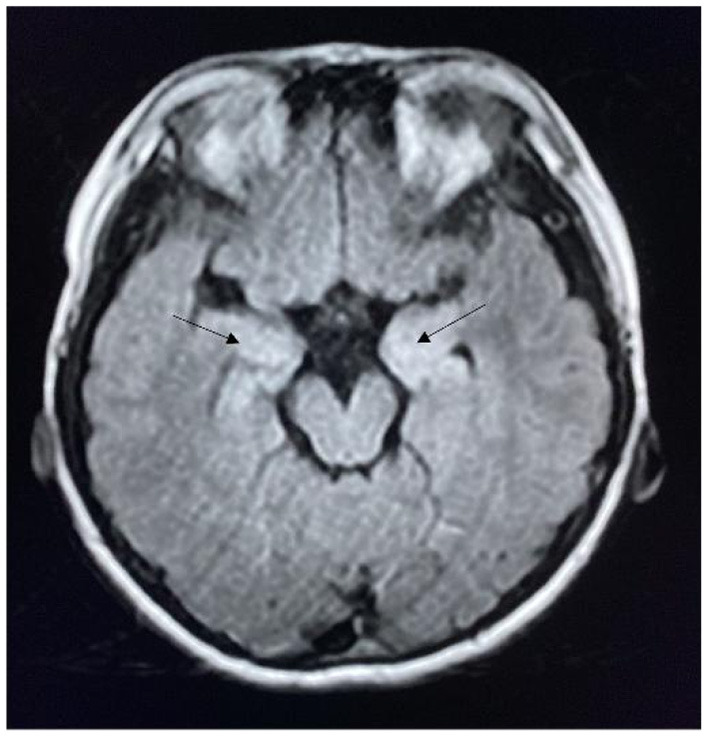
MRI of a patient with anti-NMDAR encephalitis showing bilateral hippocampal lesions (arrow).
